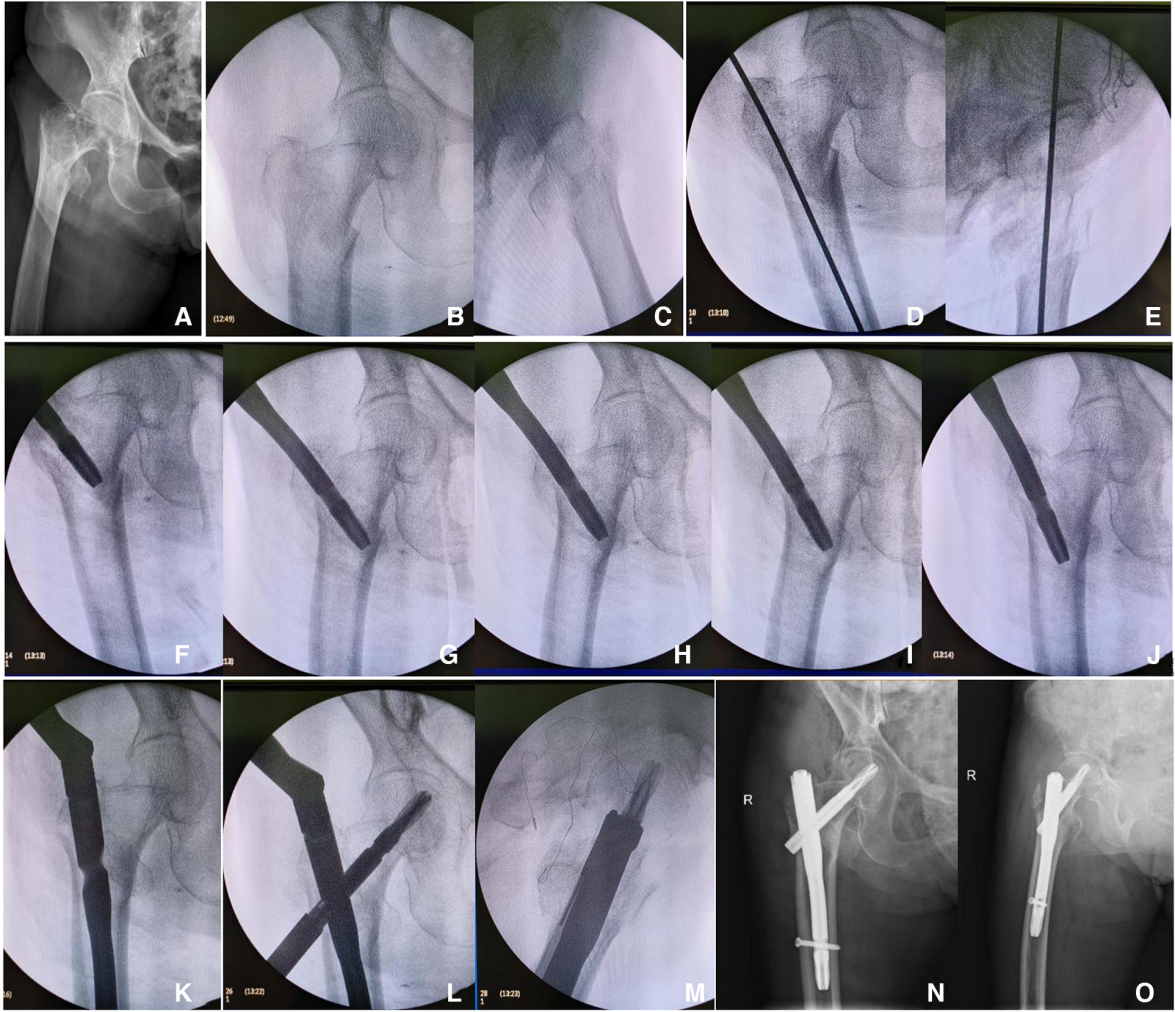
Mrs. Zhang, Female, 82 years old. (A) Preoperative x-ray in the anteroposterior position; (B,C) intraoperative confirmation by fluoroscopy on the C-arm machine of irreducible intertrochanteric femoral fracture with negative medial cortical support; (D,E) anteroposterior and lateral positioning of the nail entry point, inserting the guide wire, and preparing for proximal reaming; (F–K) intraoperative utilization of intramedullary reduction for irreducible fracture reduction, converting the negative medial cortex support to medial cortex anatomical reduction; (L,M) post-reduction fixation with PFNA; (N,O) postoperative x-ray in anteroposterior and lateral views showing anatomical reduction of the fracture.
